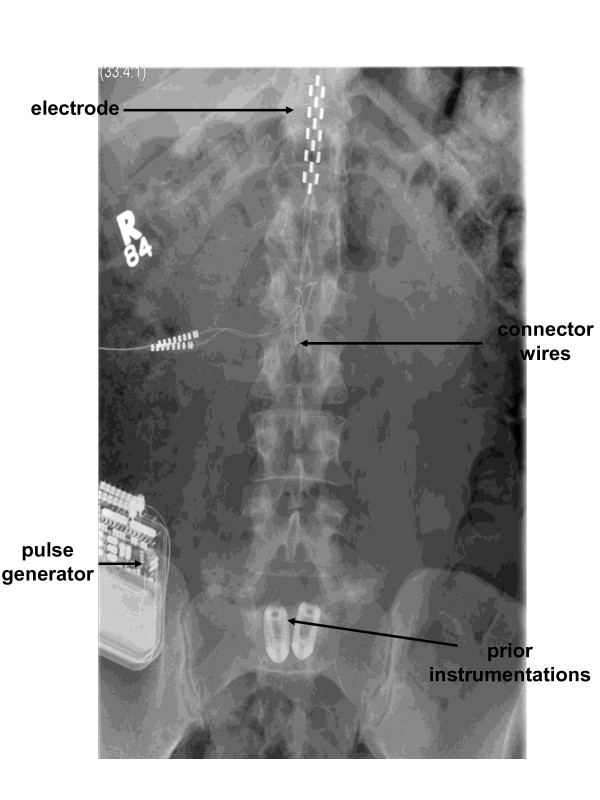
An AP X-ray demonstrating position of the epidural spinal cord stimulation electrode, connector wires, and pulse generator located in the right lower quadrant. The patient's prior instrumentation in the lumbo-sacral area is also visualized.
